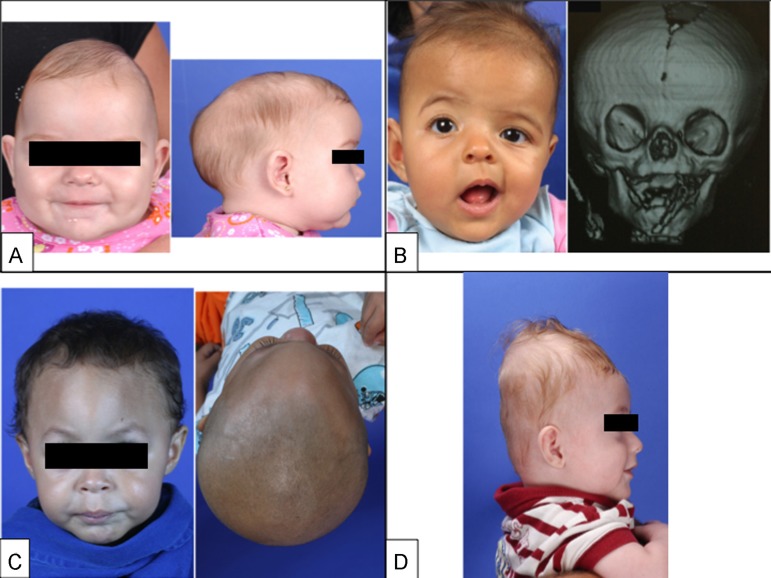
(A, Left) Frontal photograph of patient with premature fusion of sagittal suture showing the characteristic temporal pinching. (Right) Lateral photograph reveling increase in the anterior-posterior diameter of the skull (long narrow skull), the frontal bossing and occipital bulging (occipital bullet), which are the main clinical characteristics of sagittal craniosynostosis. (B, Left) Frontal photograph of patient with premature fusion of the right coronal suture showing the retrusion of the ipsilateral frontal bone fusion and compensatory contralateral bulging, asymmetry of the eyebrows, orbits, ears, nose, jaw, as well as convergent strabismus of the left eye. (Right) 3D CT reconstruction showing the premature fusion of the right coronal suture and the elevation of the ipsilateral sphenoid wing leading to an elongate orbit, recognized as the “harlequin orbit”. (C, Left) Frontal photograph of patient with premature fusion of metopic suture showing the triangular aspect of the forehead with retruded crests of the orbits bilaterally and hypoteleorbitism (approximation of orbits). (Right) Basal view revealing the triangular appearance of the skull. (D) Lateral photograph of patient with premature fusion of lambdoid suture showing the turricephalic aspect of the skull. Two-dimensional photographs and radiological documentations belong to SOBRAPAR Hospital's archives. Informed consent forms were signed by the patient's parents.
